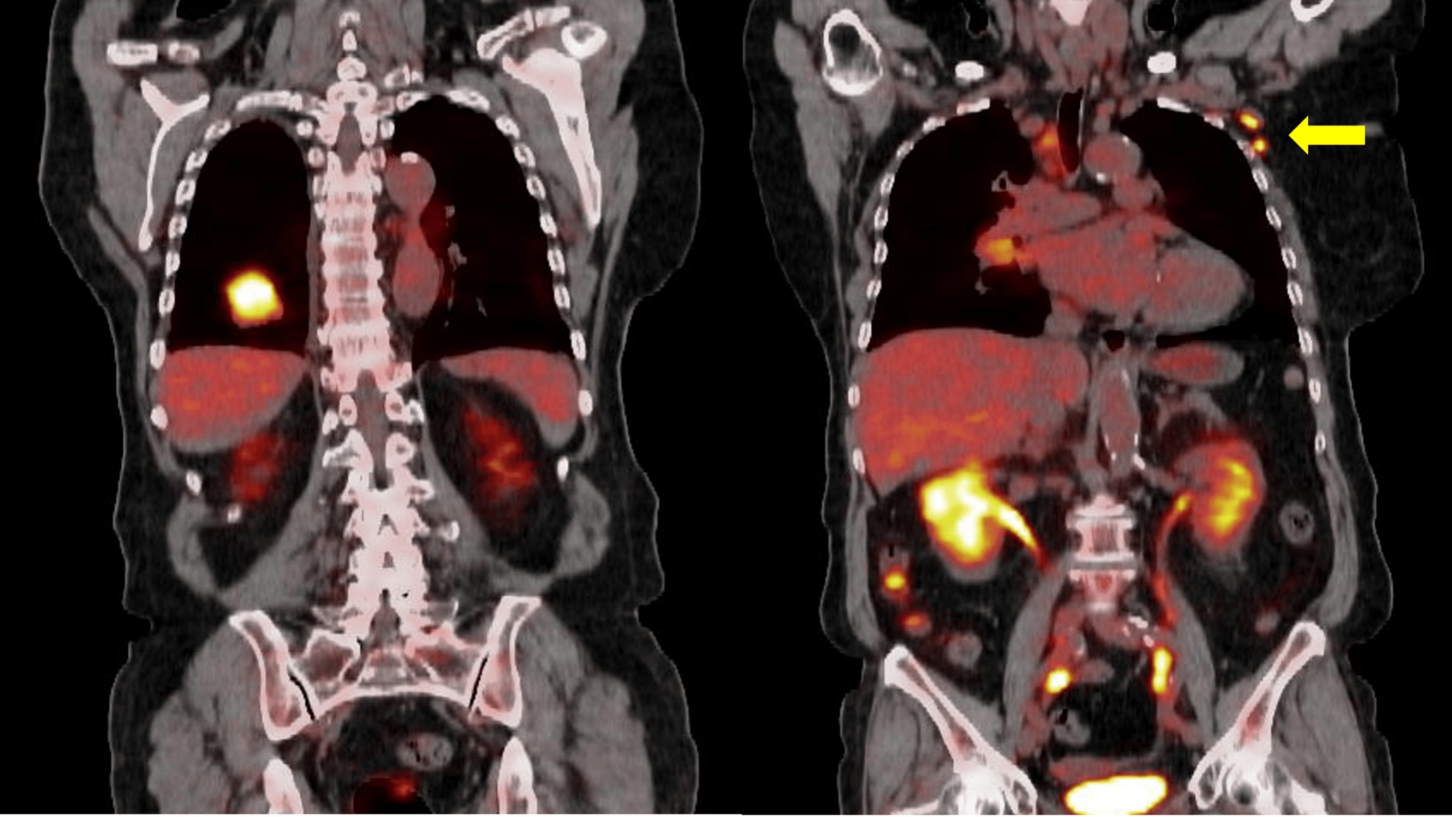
Coronal positron emission tomography/CT scan shows increased uptake of 18F-fluorodeoxyglucose to the tumor of the right lower lobe and left axillary lymph nodes (arrow).
